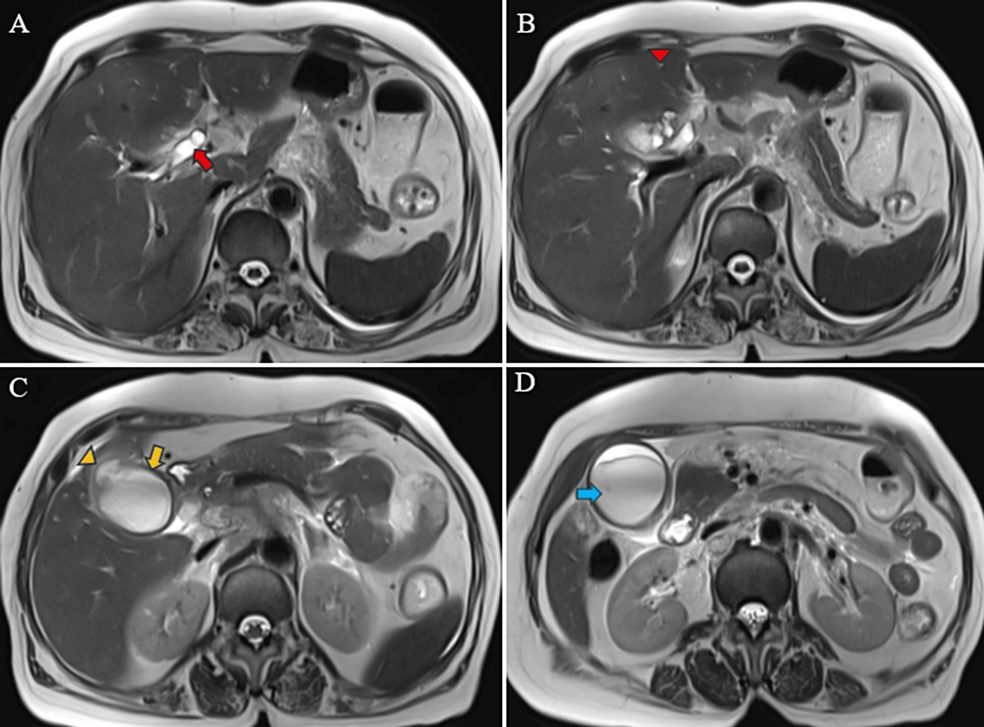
MR abdomen T2WI axial images reveal dilated right and left hepatic ducts (red arrow) with prominent intrahepatic bile radicles (IHBR) (red arrowhead) (A, B). A thickened gallbladder wall (yellow arrow), a defect in the gallbladder body region (yellow arrowhead), and a pericholecystic collection (C) are observed. Additionally, a distended gallbladder with a collection within it is seen (blue arrow) (D). MR: Magnetic resonance; T2WI: T2-weighted imaging
